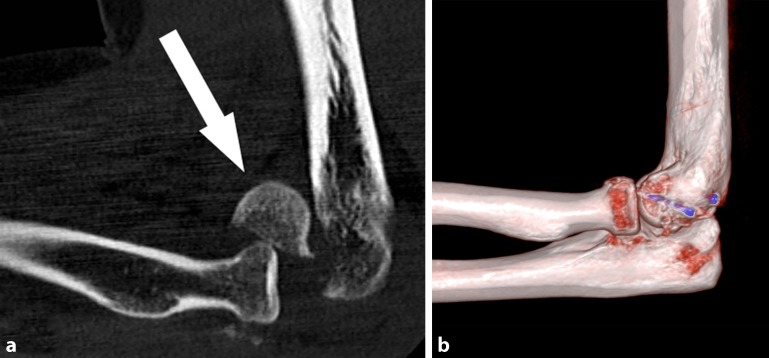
a Sagittal computed tomography reconstruction of the injured elbow. Shear fracture of the capitellum (type I) with associated anterior radial head injury (*arrow*). b After osteosynthesis with two headless screws (in posterior–anterior direction)
